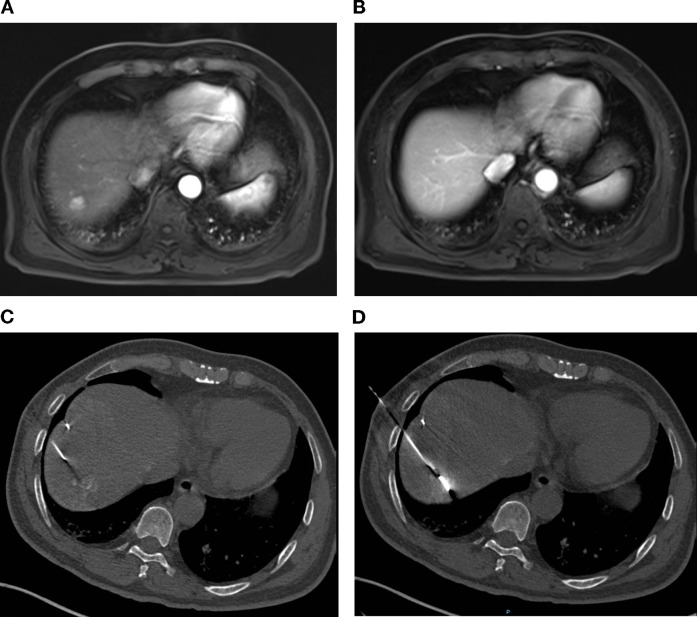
Fine needle-assisted puncture positioning technique in computed tomography-guided microwave ablation for a patient with hepatocellular carcinoma in liver segment S7. (A, B) enhanced MRI scans showed a tumor mass in liver segment S7. (C) a 22-gauge PTC fine needle was inserted near the tumor mass. (D) a microwave electrode needle was gradually inserted into the tumor according to the mark of the fine needle while the patient held their breath.
